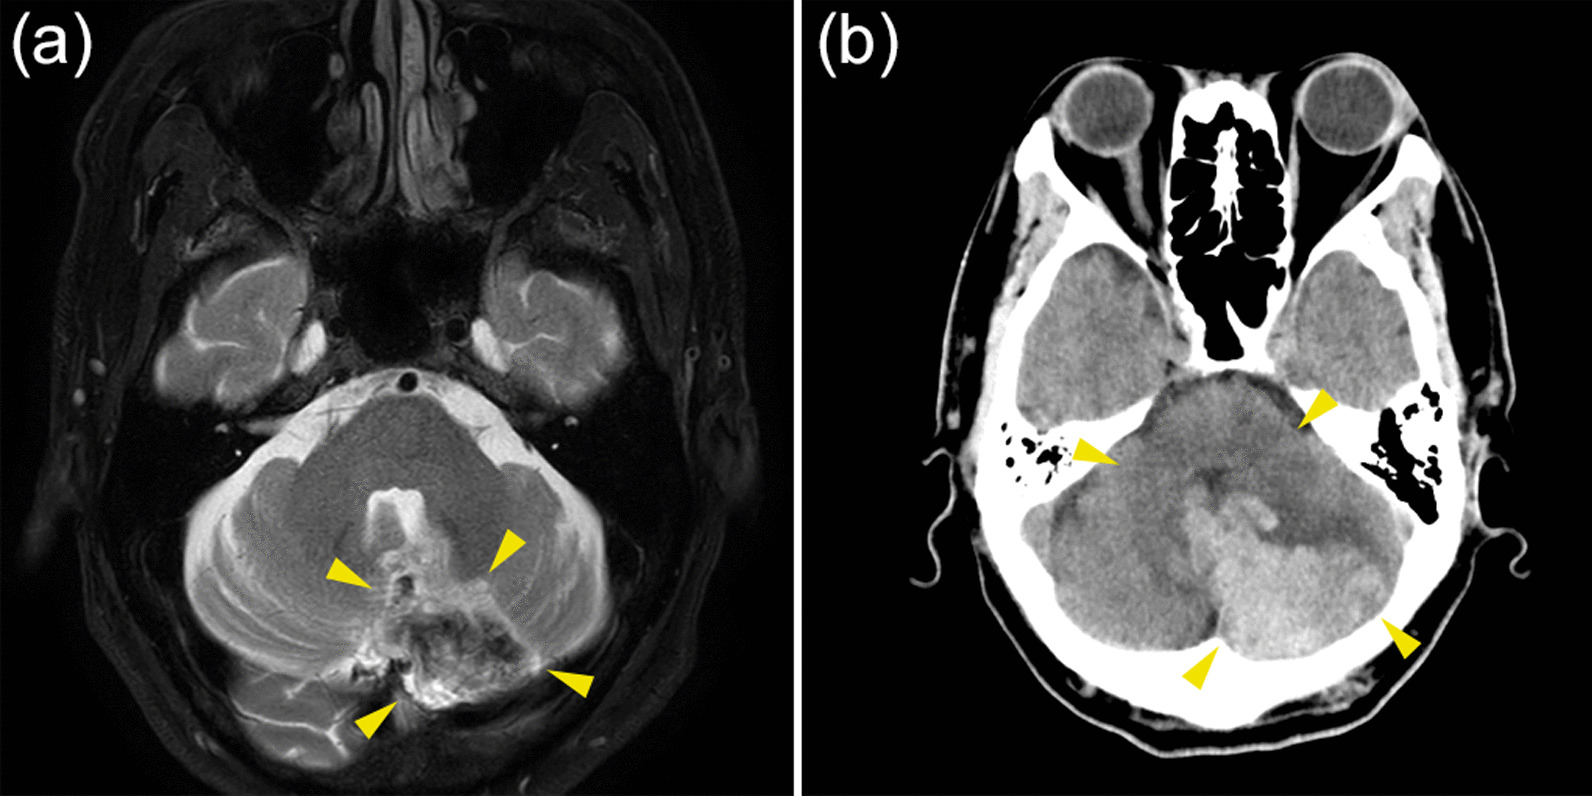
a T2-weighted magnetic resonance imaging (MRI) performed for diplopia 10 days after the second PCI. A heterogeneous low-signal area in the left cerebellar hemisphere and a high-signal area in its limbus (yellow arrowheads) in the posterior inferior cerebellar artery territory can be observed. Based on the symptoms and images, hemorrhagic cerebral infarction was diagnosed. b Three days after MRI revealed the hemorrhagic cerebral infarction, head computed tomography (CT) was performed because of the occurrence of bradykinesia. CT showed hemorrhage (high density area) and edema around it (low density area) (yellow arrowheads). The hematoma and edema have worsened and compressed the fourth ventricle
